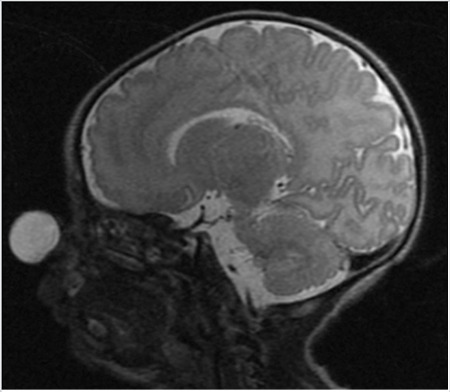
Sagittal T2 MRI view of the lesion MRI: Magnetic resonance imaging
